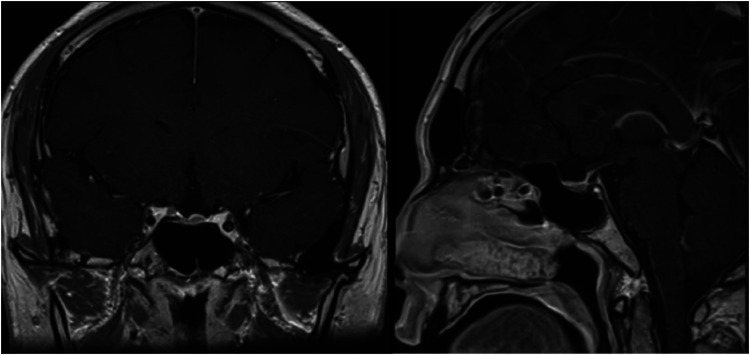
Post-operative MRI after 18 months.
